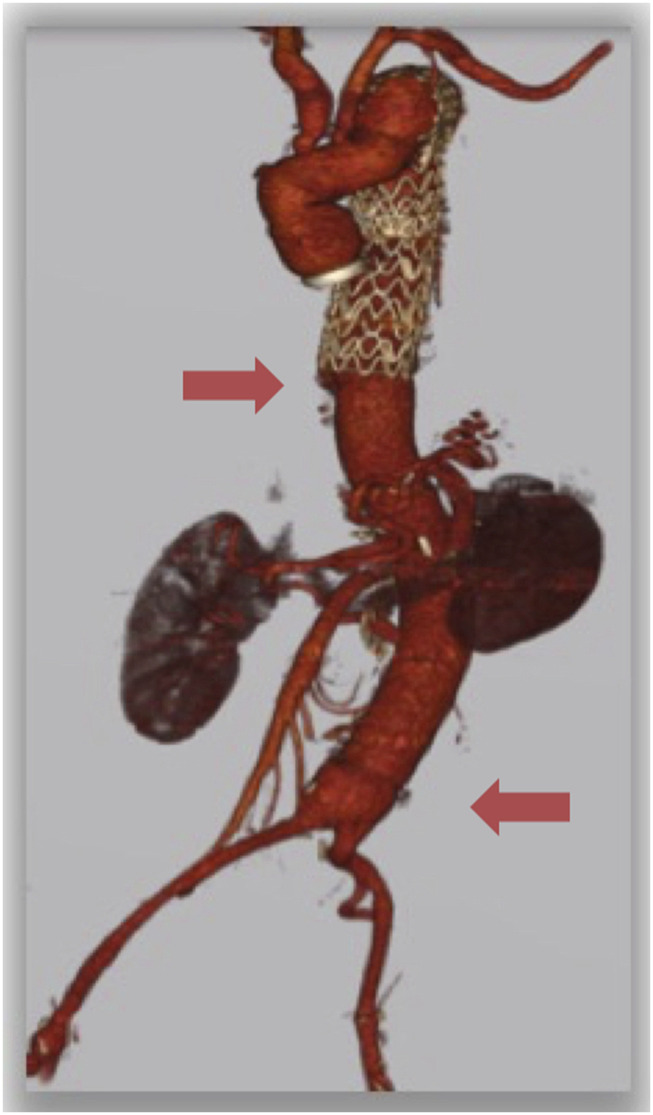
Follow-up CT scan showing good results of TAAA open repair with partial excision of the thoracoabdominal endograft, bypass with a 30-mm multi-branched “Coselli” aortic graft (*Vascutek, Renfrewshire, Scotland, UK*) and patency of all visceral vessels bypasses and no pathological findings at the level of the thoracic endografts and anastomosis.
